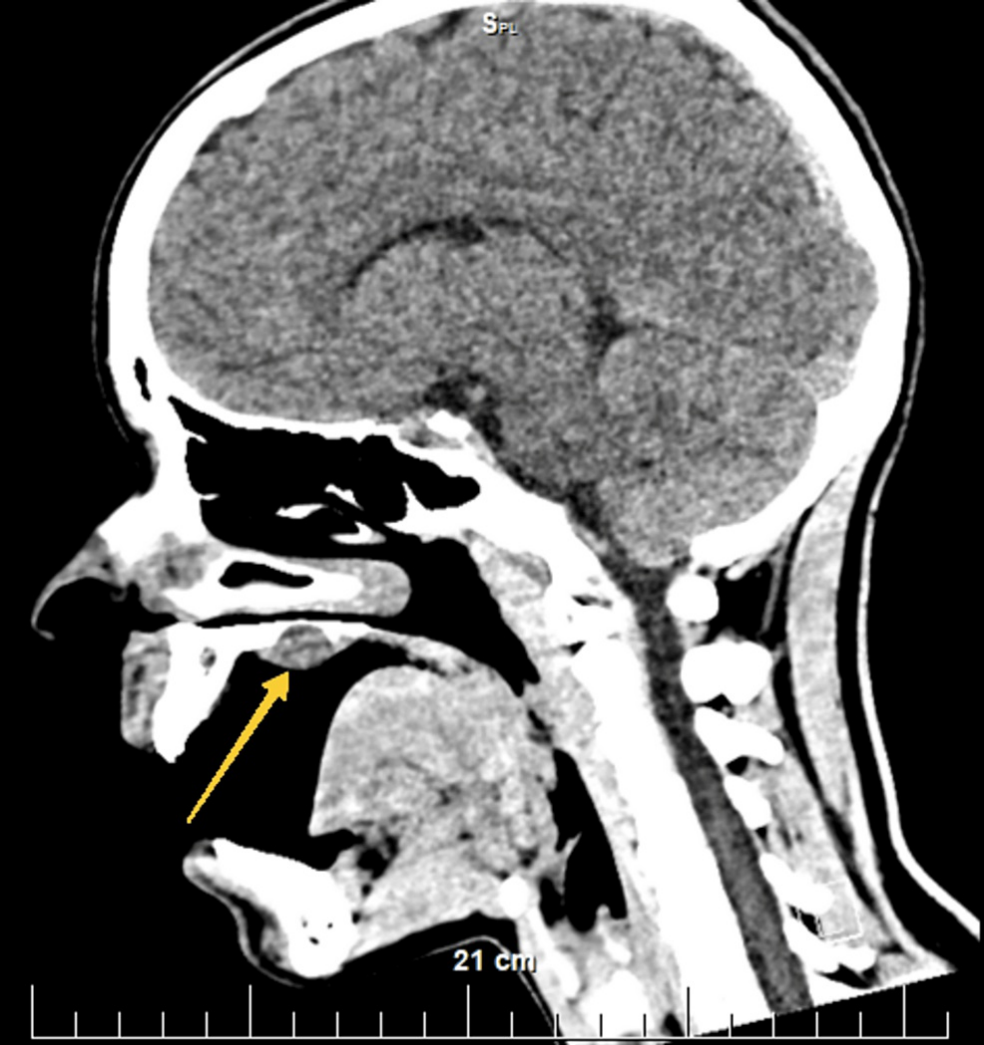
Sagittal section of a native head CT. A yellow arrow indicates the location of the hard palate lesion on the right. Above it, the thinning of the bony plate of the hard palate can be seen
